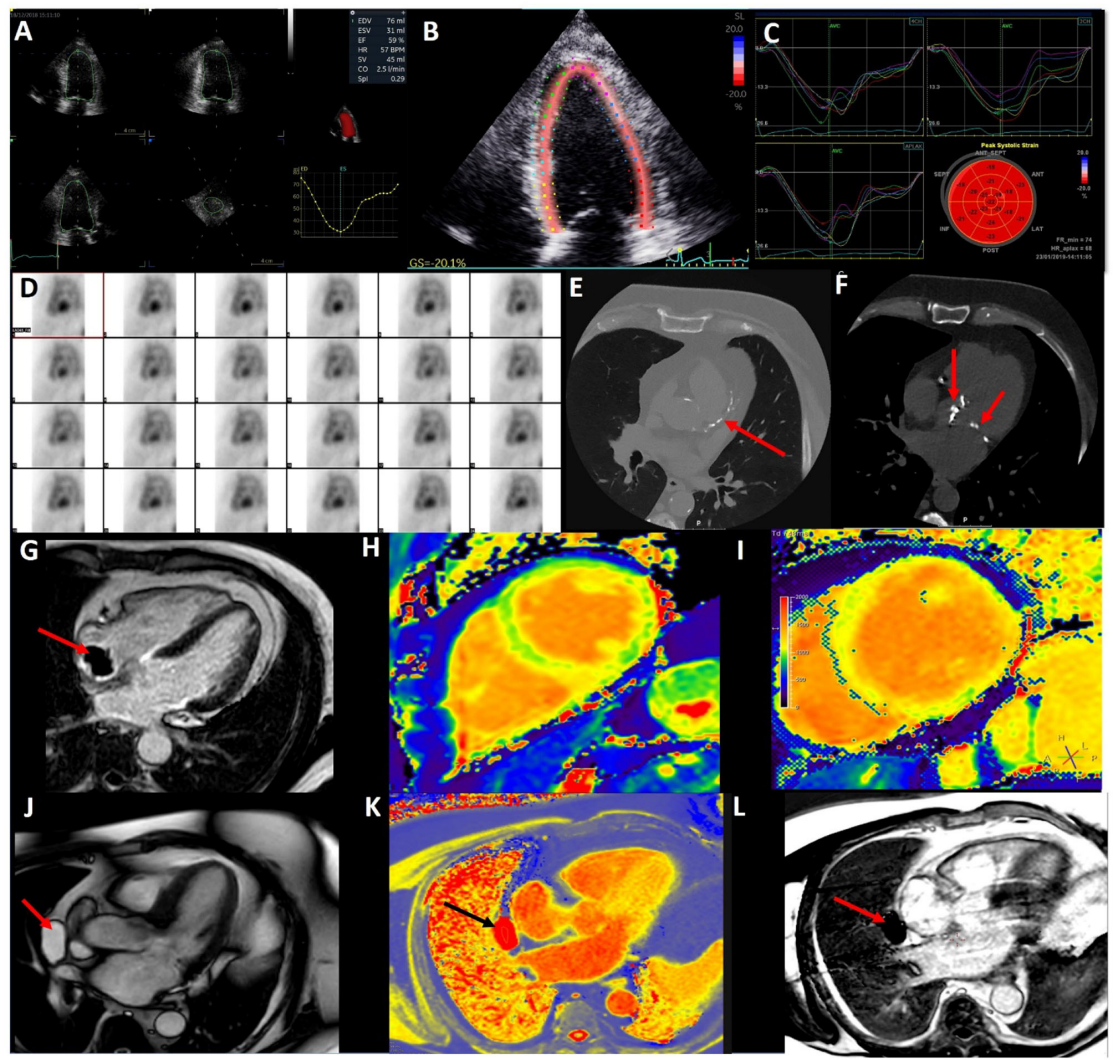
Multi-modal imaging in cardio-oncology. (A) 3D echocardiography to accurately calculate left ventricular volumes and ejection fraction. (B) 2D Speckle Tracking Echocardiography of the left ventricle (4 chamber view) for Global Longitudinal Strain. (C) 2D Left Ventricular Global Longitudinal Strain curves from 4, 2, and 3 chamber views. (D) Nuclear medicine—Multi-gated blood pool imaging to determine LVEF. (E) CT coronary angiogram demonstrating coronary artery calcium in the left anterior descending artery in a lymphoma survivor. (F) CT coronary angiogram demonstrating radiotherapy related aortic and mitral valve calcification in the same patient. (G) Cardiac Magnetic Resonance (CMR) late gadolinium enhancement with long T1 inversion time demonstrating a thrombus (red arrow) on the end of a Hickman's line in a cancer patient. (H) Normal CMR T1 map (green is normal myocardium). (I) T1 map showed elevated T1 times of the left ventricle in myocarditis. (J) CMR 3Ch cine demonstrating a pericardial mass (red arrow). (K) CMR T1 map highlight the pericardial mass is fill with fluid (black arrow). (L) CMR LGE with long T1 inversion time demonstrating mass (red arrow) is avascular with no enhancement.
